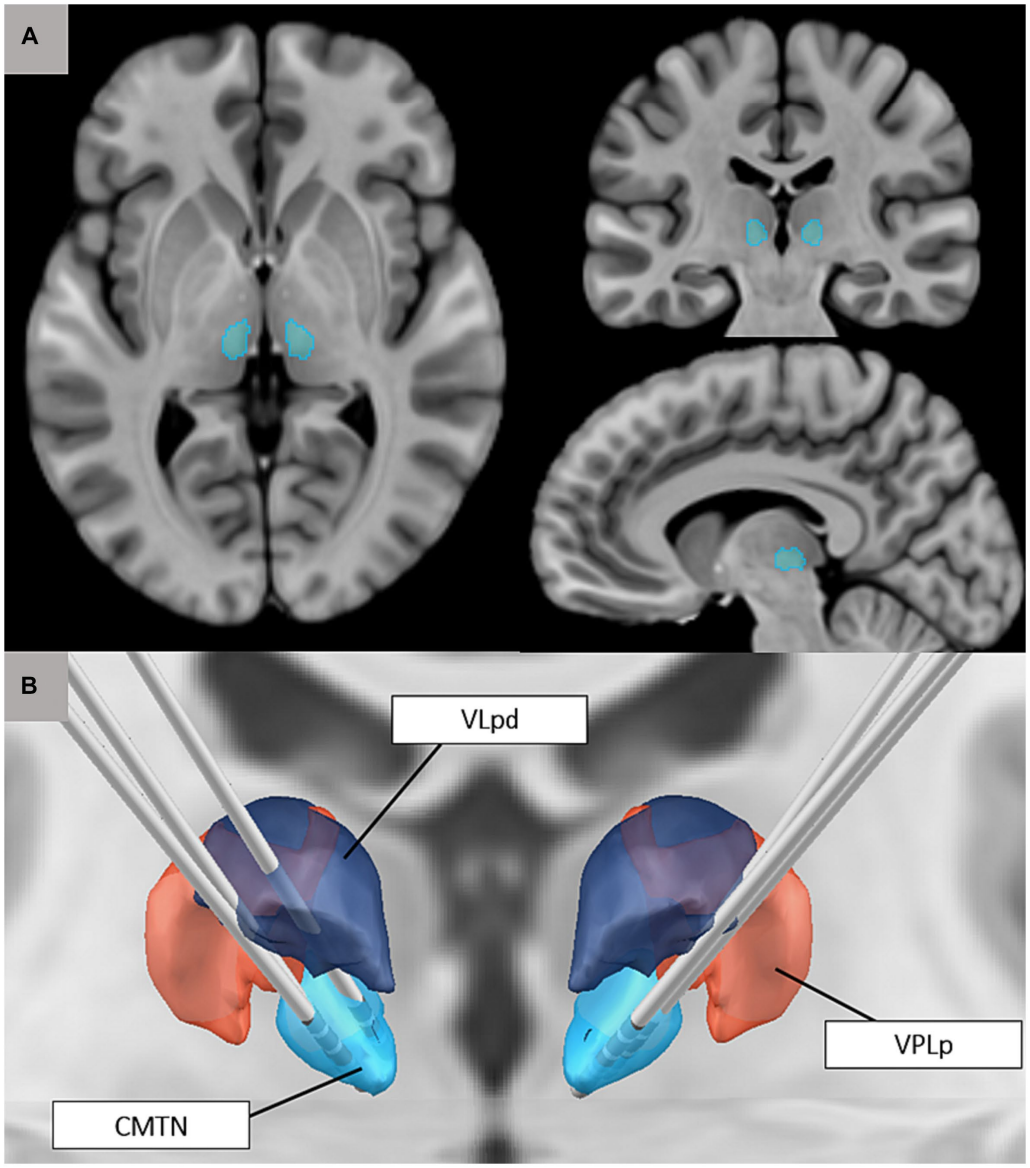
(A) Visualization of the centromedian thalamic nucleus based on the Morel thalamic atlas on adult anatomy (T1-weighted MNI template image). (B) 3D rendering of the CMTN (blue) in relationship to ventral posterolateral nucleus (VPLp; red) and posterior dorsal part of the ventral lateral nucleus (VLpd; purple). Also pictured are reconstructions of bilateral CM-RNS trajectories for three cases (27).
